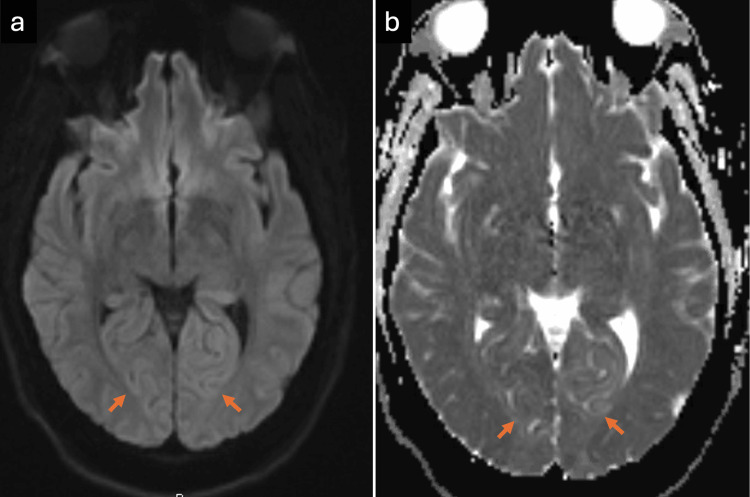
MRI of the brain (a) DWI and (b) apparent diffusion coefficient sequences show no diffusion restriction (arrows) in the corresponding occipital lobe regions, consistent with vasogenic edema. DWI: Diffusion-weighted imaging
